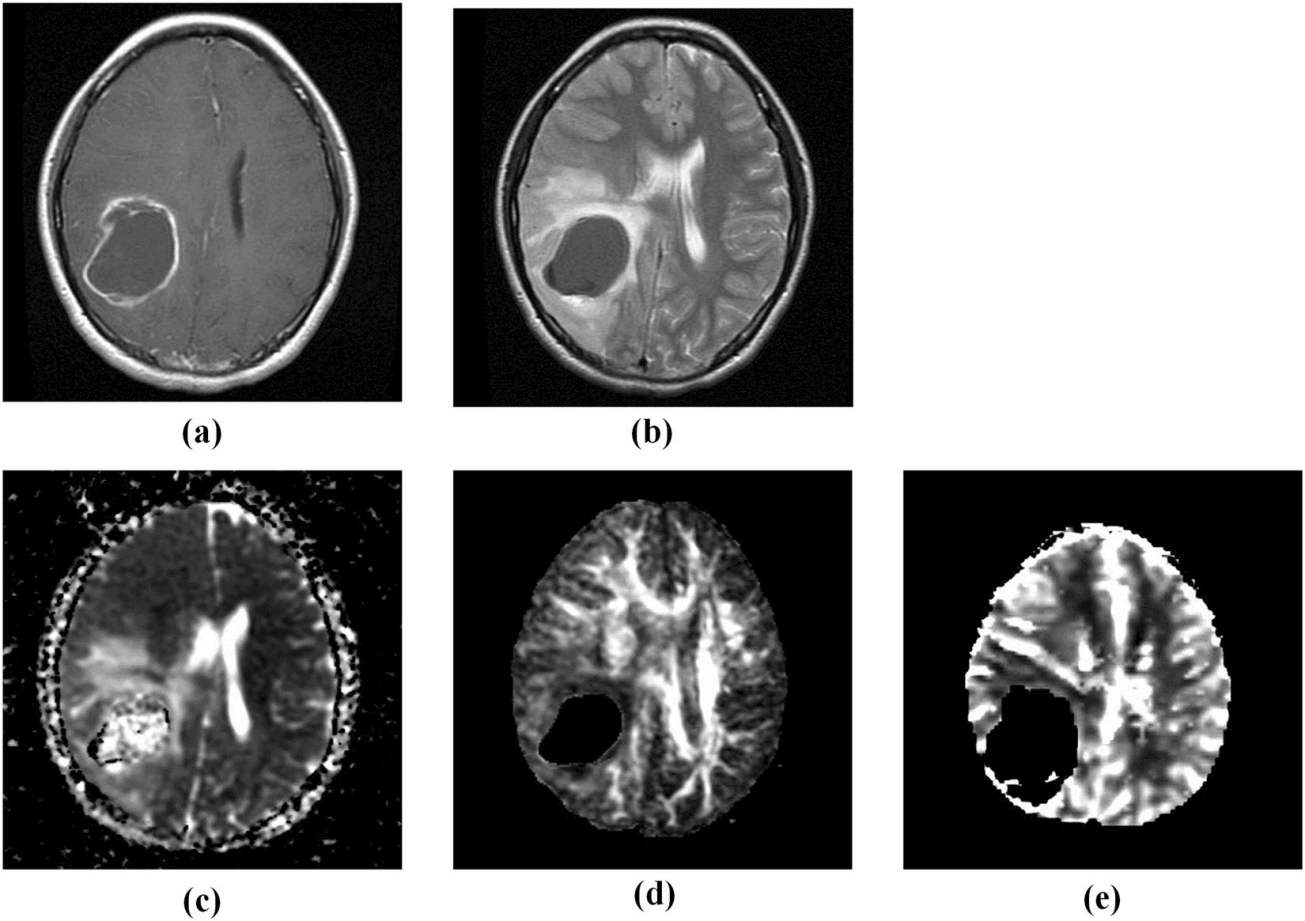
Multimodal images of a patient with glioblastoma multiforme (GBM). (a) T1-weighted contrast-enhanced image, (b) T2-weighted image, (c) apparent diffusion coefficient (ADC) map, (d) fractional anisotropy (FA) map, (e) relative cerebral blood volume (rCBV) map. The appearances of tumor in anatomical and functional images are different from each other.
